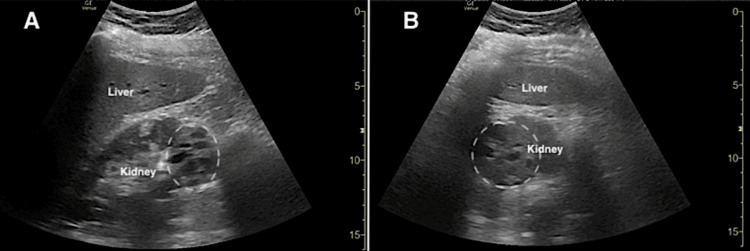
Point-of-care ultrasound demonstrating a renal mass (dotted circle) of the right kidney in longitudinal (A) and transverse (B) planes.
